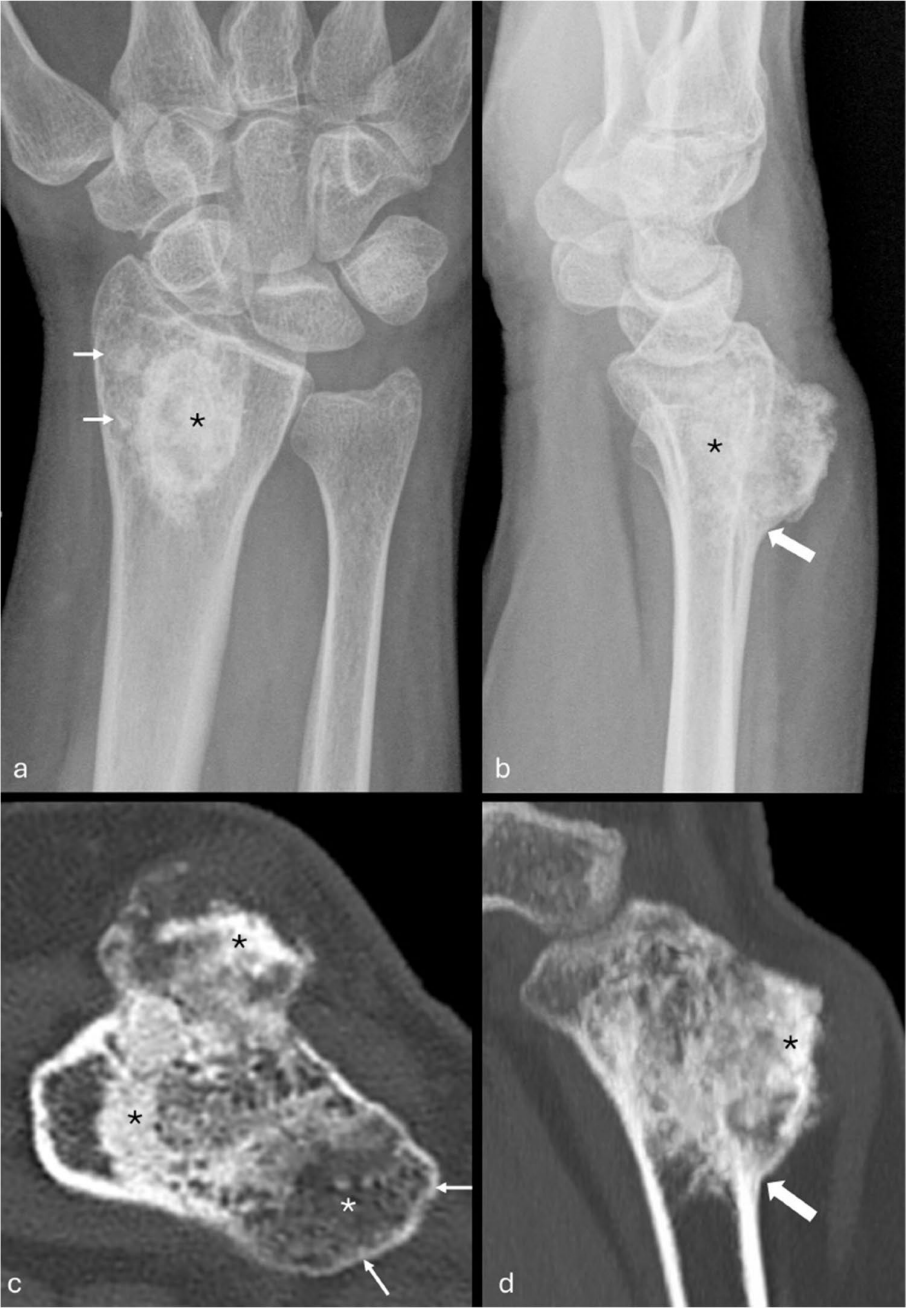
Case 1. Frontal (a) and lateral (b) radiographs of the right wrist. A well-defined focus of increased density is projected over the distal radius (asterisk, a) with further faint densities laterally (arrows). A heavily ossified mass protrudes dorsally, but increased density projected over the distal radius suggests intramedullary tumor (asterisk, b). The dorsal cortex is “split” at the proximal aspect of the tumor suggesting intracortical growth (block arrow, b). Axial (c) and sagittal (d) CT reconstructions of the right wrist. There is heterogeneous intramedullary sclerosis and an ossified exophytic mass (black asterisks). Tumor at the radial/lateral aspect of the radius shows only faint sclerosis (white asterisk, c) and there is adjacent cortical thinning (thin arrows, c). Sclerosis extends from the bone into the protuberant mass, and there is intracortical growth proximally (block arrow, d)
